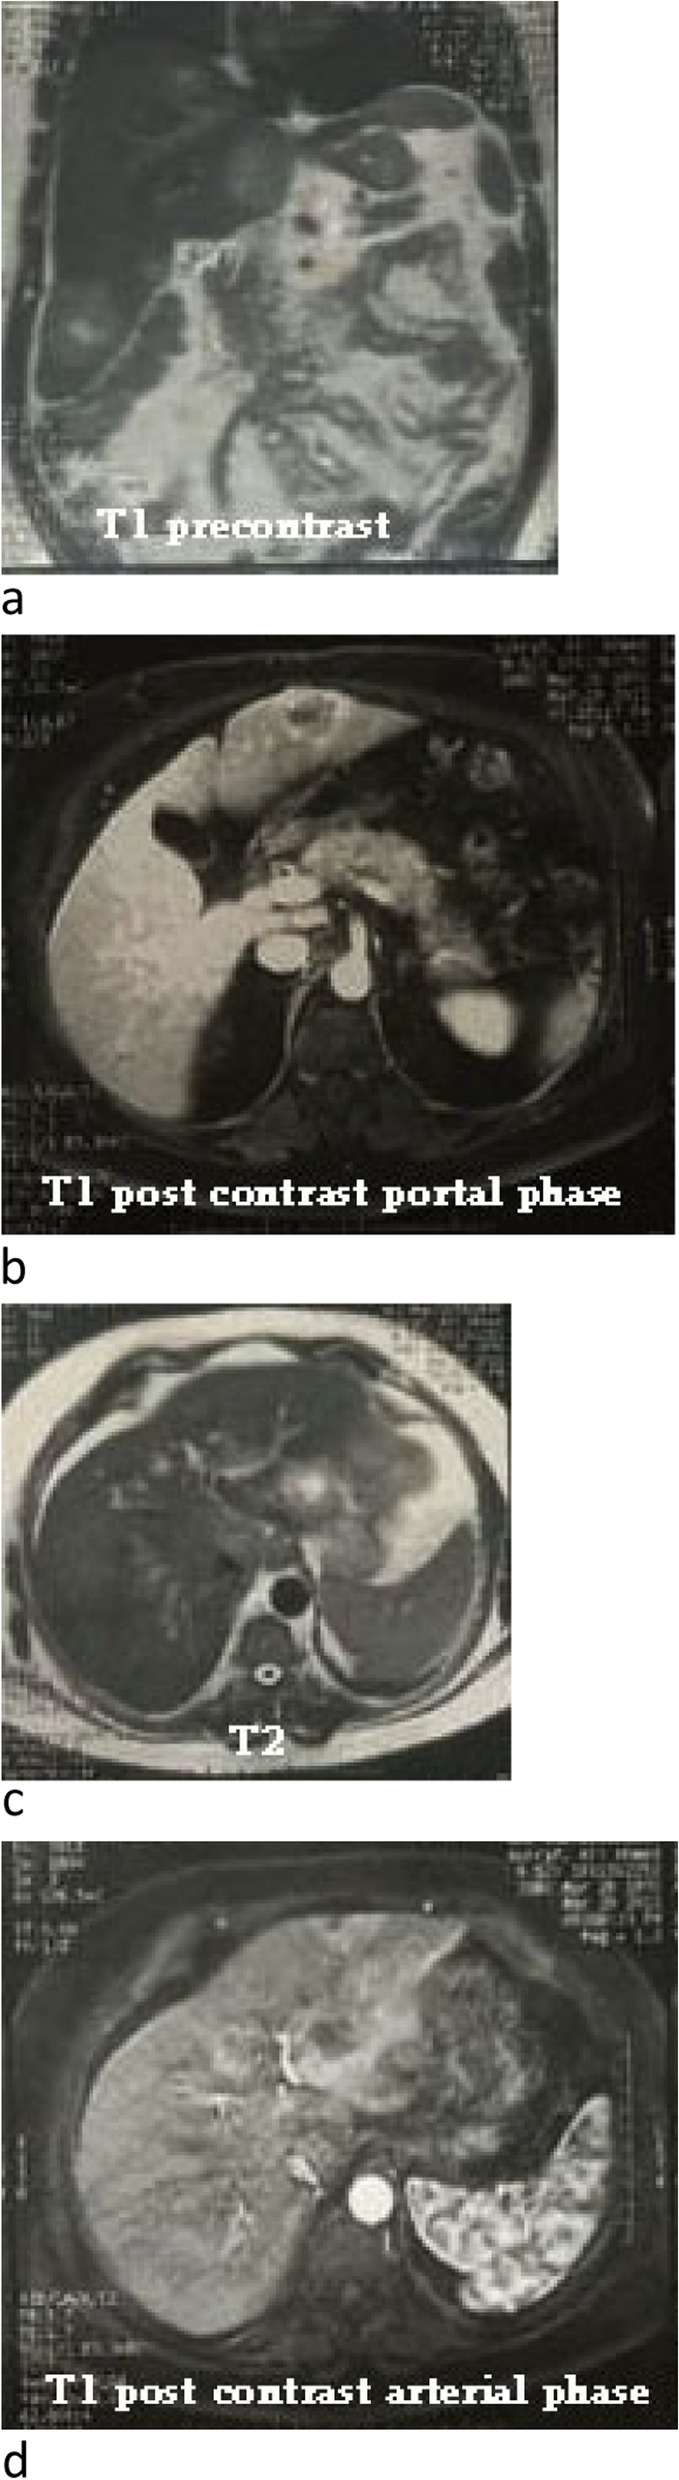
Abdominal MRI. Heterogeneous low T1 and higher T2 signal with some element of diffusion restriction along its periphery
